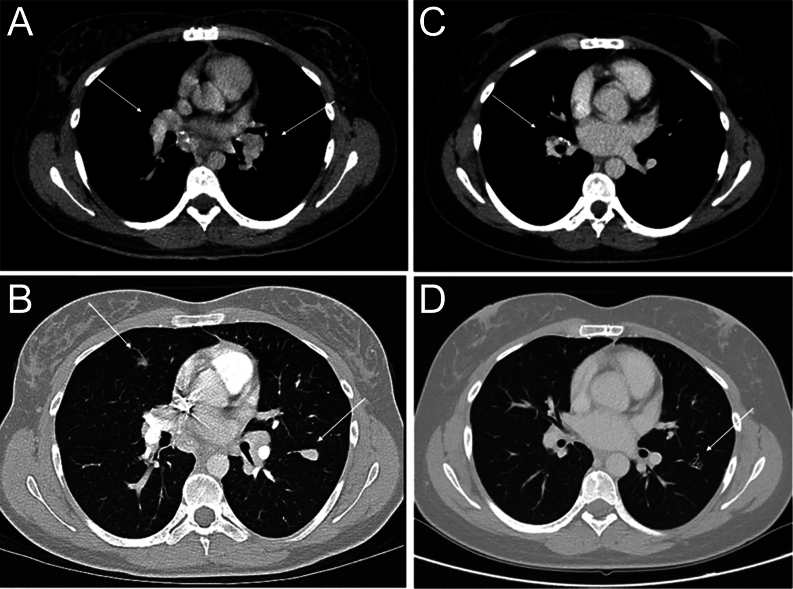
Serial CT scans showing radiological response to selpercatinib. Axial-view CT scan of the chest demonstrates bulky bilateral hilar lymphadenopathy (A) and bilateral pulmonary nodules (B) and subsequent radiological response (C, D) 3 months after commencing selpercatinib.
